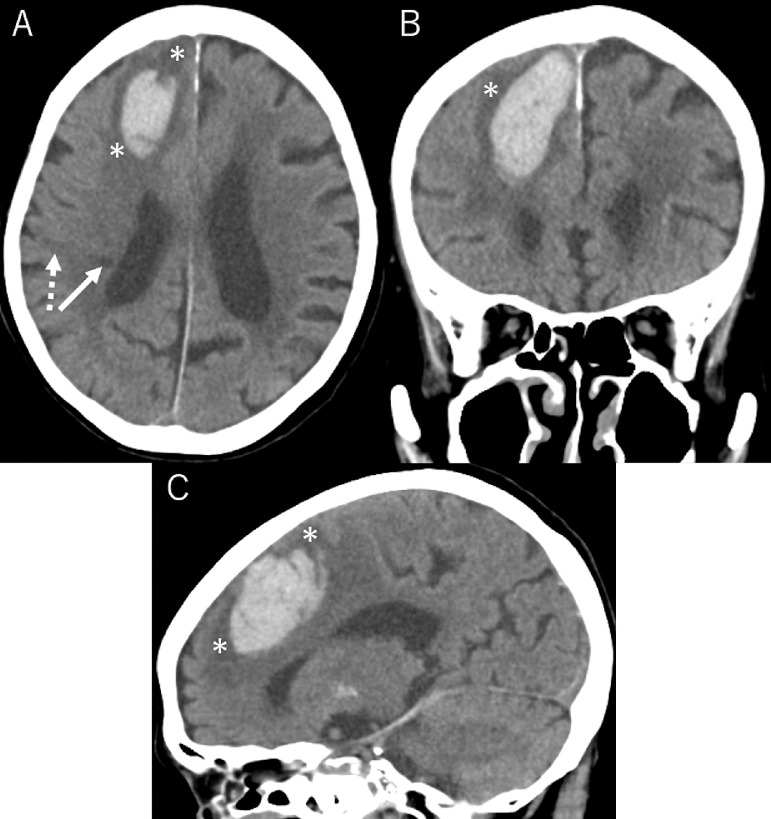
Non-contrast axial (A), coronal (B), and sagittal (C) computed tomography scans showing a subcortical hemorrhage, 38 × 45 × 47 mm in maximal dimension, in the anterior part of the right frontal lobe, accompanying perilesional cerebral edema (A-C*,* asterisks), in addition to a foggy low-density area in the right frontal lobe (A*,* dashed arrow), adjacent to the previous infarction appearing as a low-density spot (A*,* arrow).
